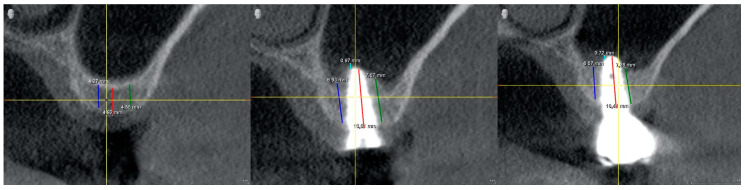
Cross-sections of the CBCT with the measurements made at different moments of the study (T0, T1, and T2).
